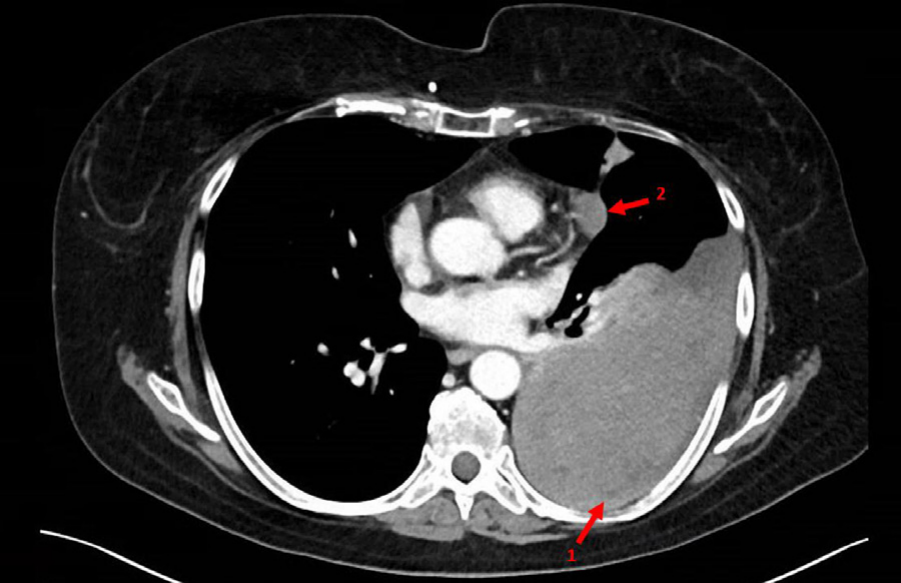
Chest computed tomography one month after presentation; growing pleural mass in the left lower lobe (arrow 1) and a new pericardial mass left (arrow 2).
